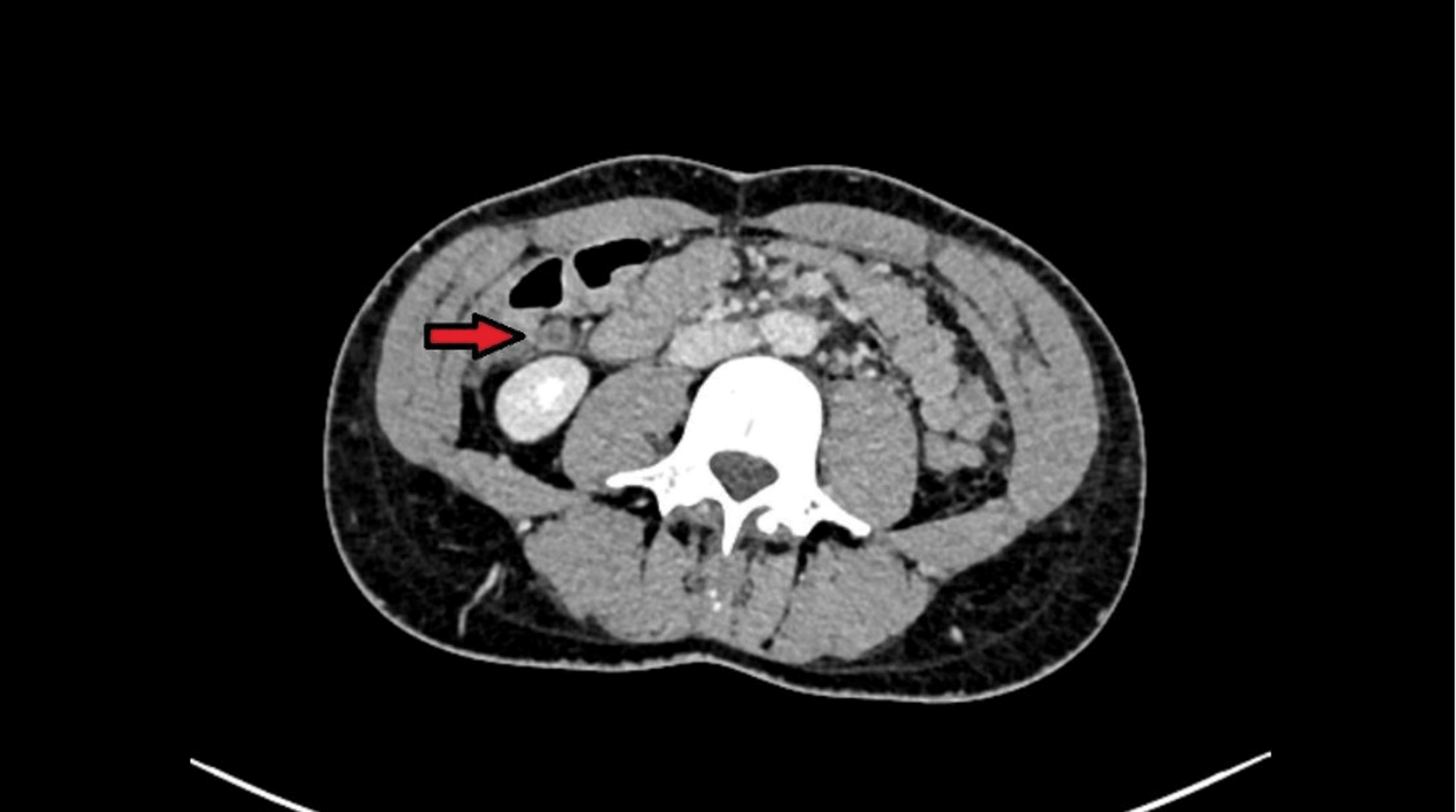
Axial abdominal CT scan image showing distended appendix with an enhancing wall and mild peri-appendiceal fat stranding.
